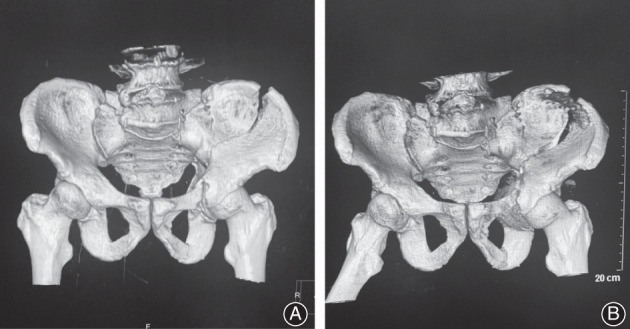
A 63‐year old male patient with HE‐PFs. (A) CT showed bilateral acetabulum, bilateral superior and inferior ramus of pubis, right sacrum, and iliac crest fractures. (B) Another pelvic radiography of the same patient was taken 1 week after open reduction and internal fixation
